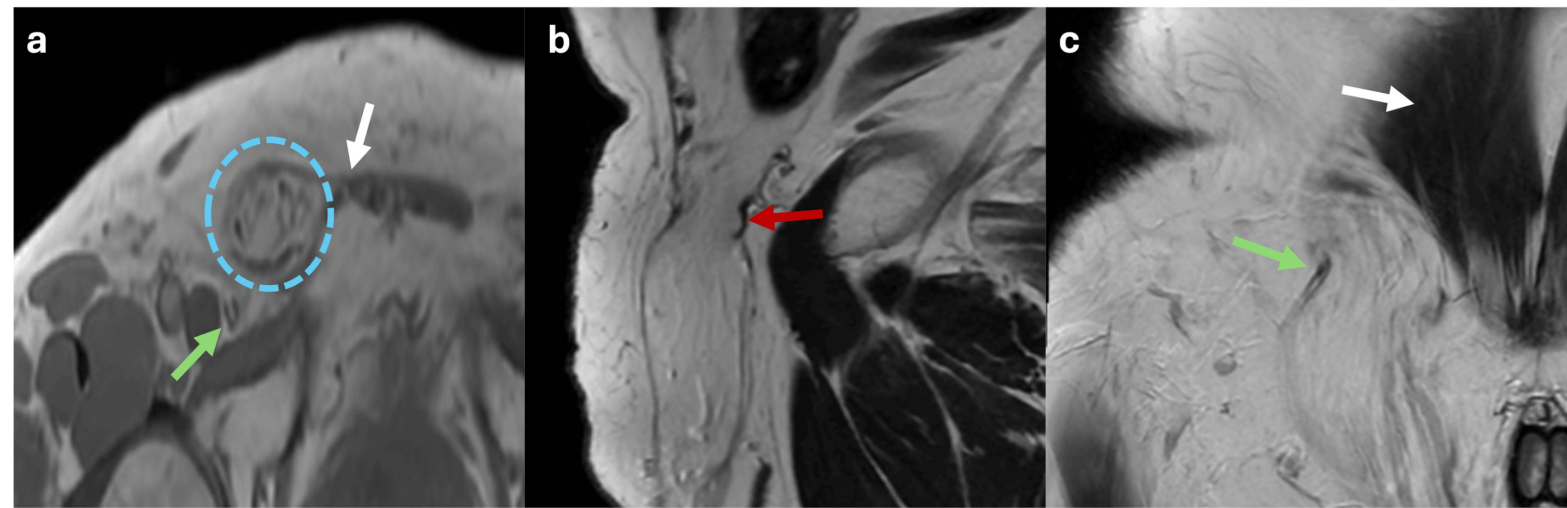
Axial, sagittal and coronal abdominal MRI in T1 and T2 sequences showing a direct inguinal hernia Axial T1 (a), sagittal and coronal T2 (b, c) MRI showing a hernial sac containing fat (blue dotted circle) positioned medial to the inferior epigastric vessels (green arrows), superior to the inguinal ligament (red arrow), and lateral to the rectus abdominis muscle (white arrows) within the Hesselbach’s triangle, consistent with a direct inguinal hernia. The figure was created by the authors.
